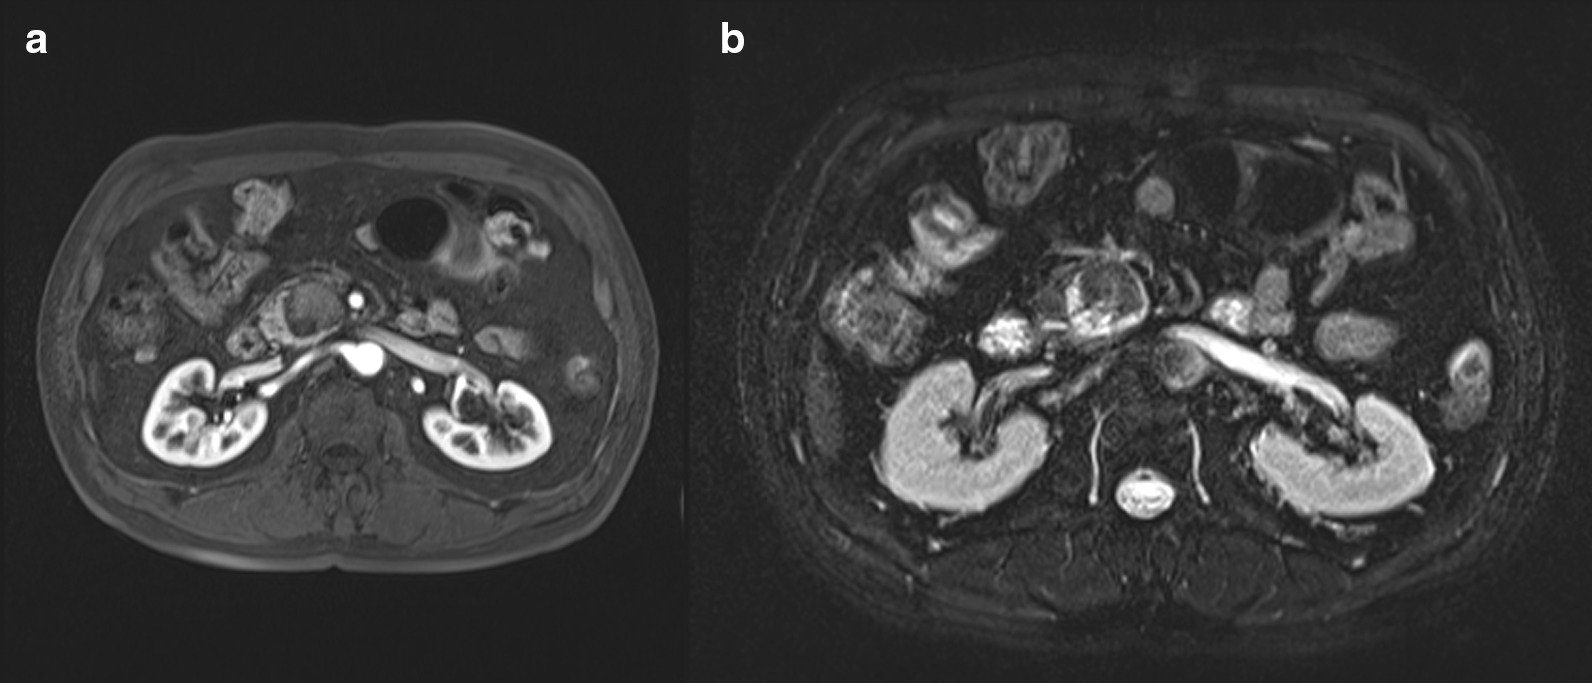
Magnetic resonance imaging showing a cystic lesion with low signal intensity (SI) and high SI focus on T1WI (a) and high SI with low SI focus on T2WI (b)
